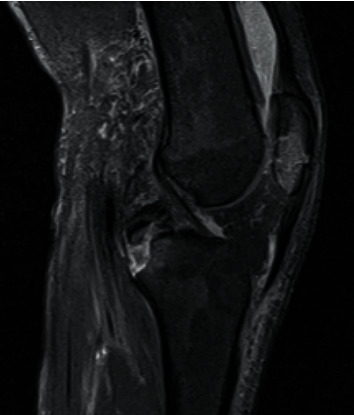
MRI (fat-saturated T2-weighted) lateral view showing a displaced avulsion fracture of PCL at tibial attachment.
